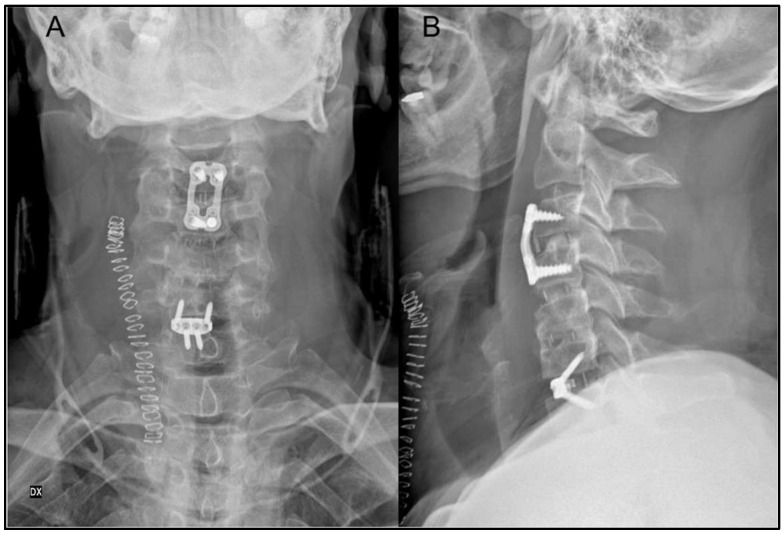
(A,B) Postoperative cervical radiographs (coronal (A), lateral (B)) showing ACDF at C3–C4 and C6–C7. The prior anterior plate from the C4–C6 construct was removed (solid fusion) to facilitate the new procedures. At C3–C4, a cage plus anterior plate was used due to intraoperative hypermobility; at C6–C7, a screw-anchored standalone cage was selected given lower segmental mobility.
